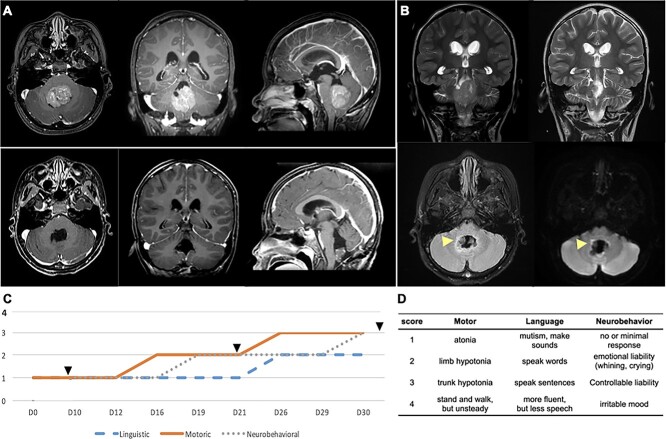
Surgical resection and postoperative course of pCMS. A) The pre- and postoperative MRI showed complete resection of medulloblastoma located at fourth ventricle via a midline trans-lower vermian approach. B) A small high signal area (white arrowhead) noted on the postoperative coronal T2-weighted imaging, axial T2 FLAIR, and DWI sequence, indicating an edema of SCP. C) A time scale of the dynamic improvement of 3 domains of symptoms for pCMS was recording according to a scoring system modified from Gudrunardottir et al.,^1^ which categorized the symptomatology of pCMS into motoric, linguistic, and neurobehavioral domains. PET studies have been arranged along this time course (black arrowhead). A score of 1–4 is graded according to the severity of symptoms within each category (D). (High-resolution figure in Supplementary Files.)
